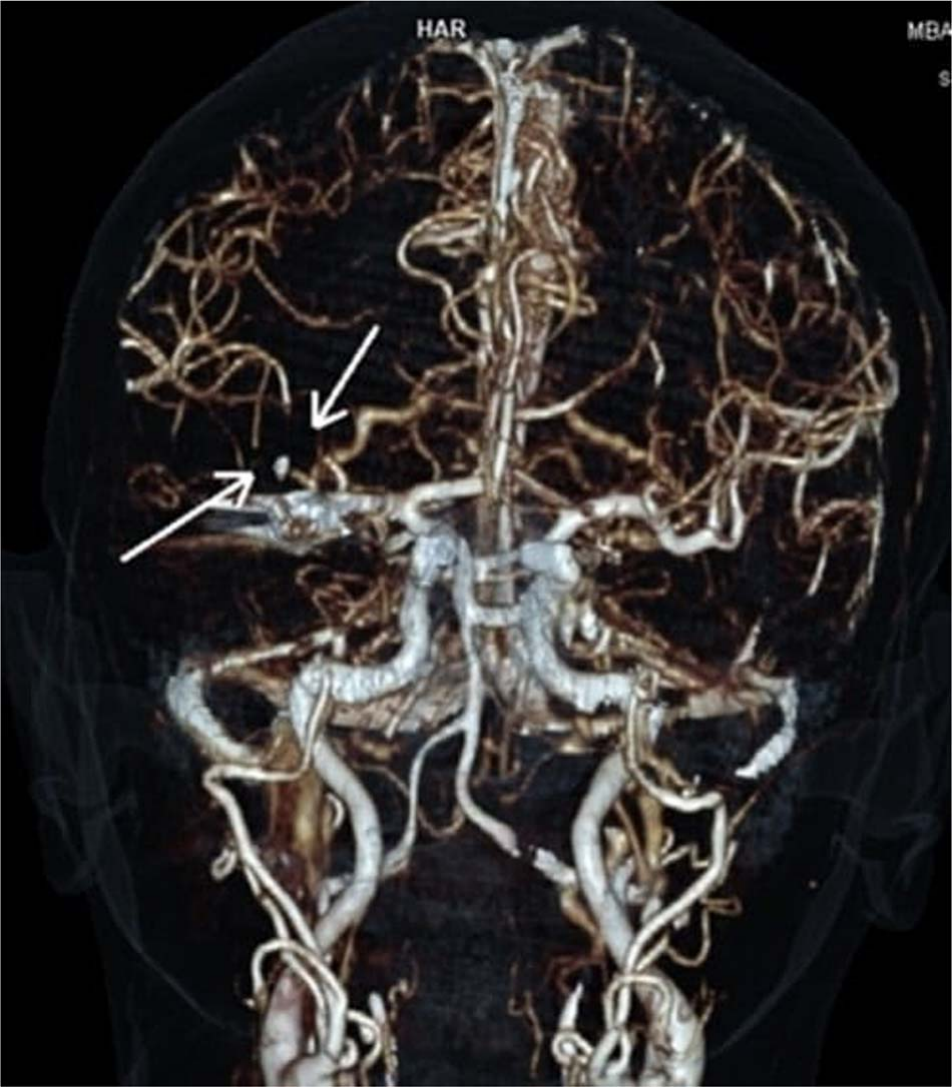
CTA reconstruction indicating proximal occlusion of M2 segmental branches of MCA (arrows).
